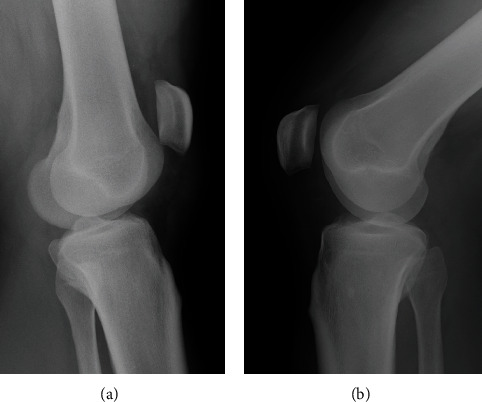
Preoperative lateral radiographs of the (a) left knee and (b) right knee.
